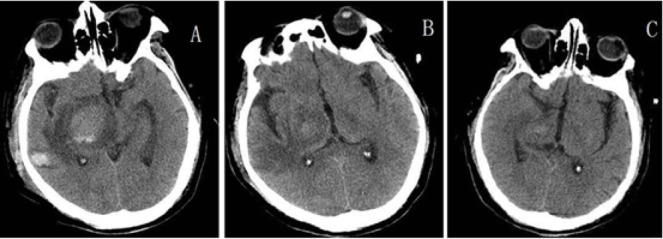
CT scan showed the right basal ganglia and right temporal lobe hemorrhage with edema (May 29^th^) A：Brain CT scan showed the right basal ganglia flake slightly higher density, CT value of about 32U, surrounding low-density, right temporal lobe low-density, the intracranial brain parenchyma density was not significantly abnormal changes. Midline slightly shifted to the left. (June 14^th^) B and (June 27^th^) C: Brain CT scan image is about similar to the image in 5.29, bleeding fade, edema reduced.
